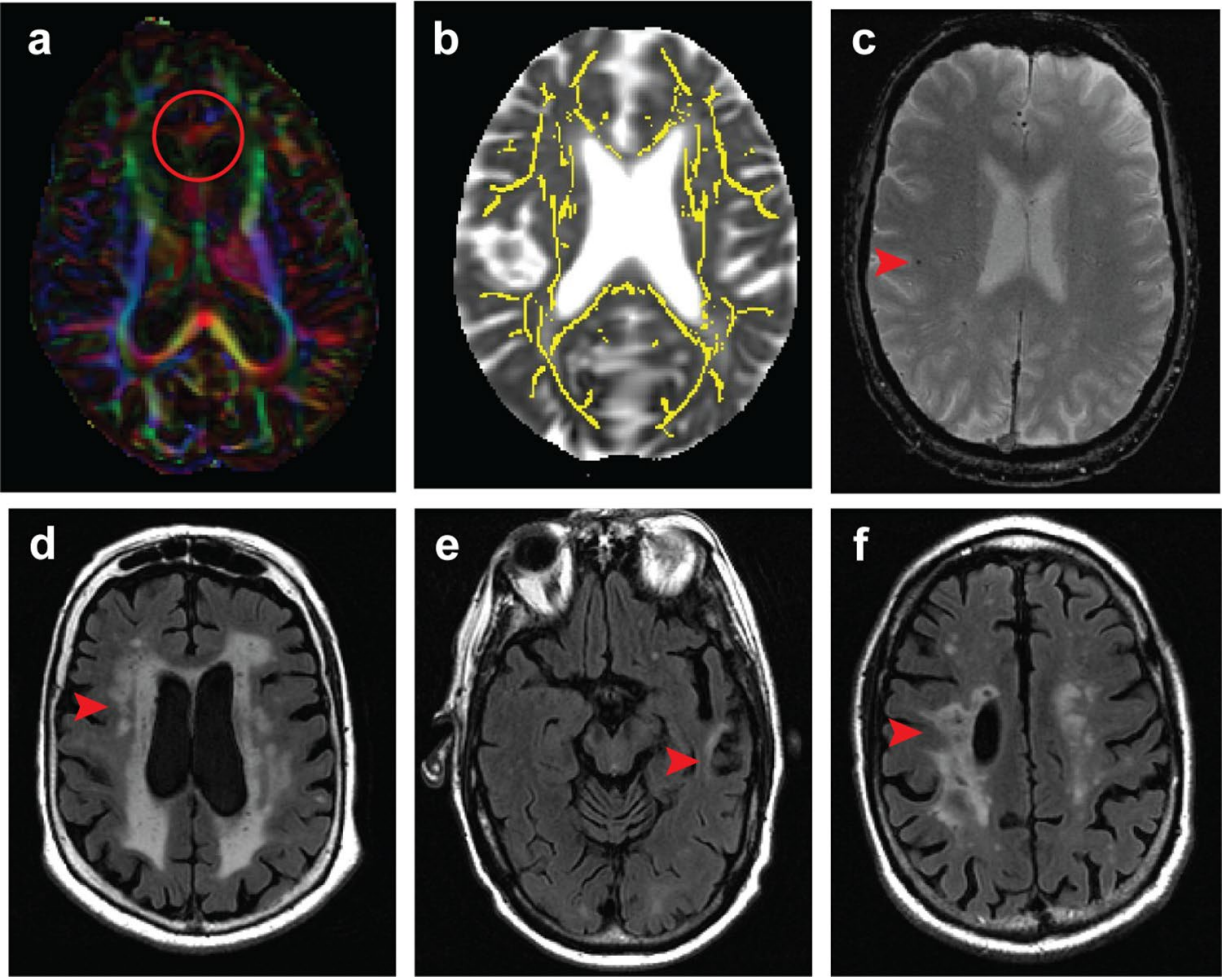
Measured CVD-related changes (a) dMRI seen as a fractional anisotropy map color map demonstrating changes in the genu of the corpus callosum measures as dMRI marker; (b) mean diffusivity image with peak skeletonized median diffusivity overlaid in red to yellow color scale; (c) T2-weighted MRI show cerebral microbleeds; (d) white matter intensities on FLAIR MRI. Both (e) cortical infarcts and (f) subcortical infarcts seen on FLAIR are demonstrated here
